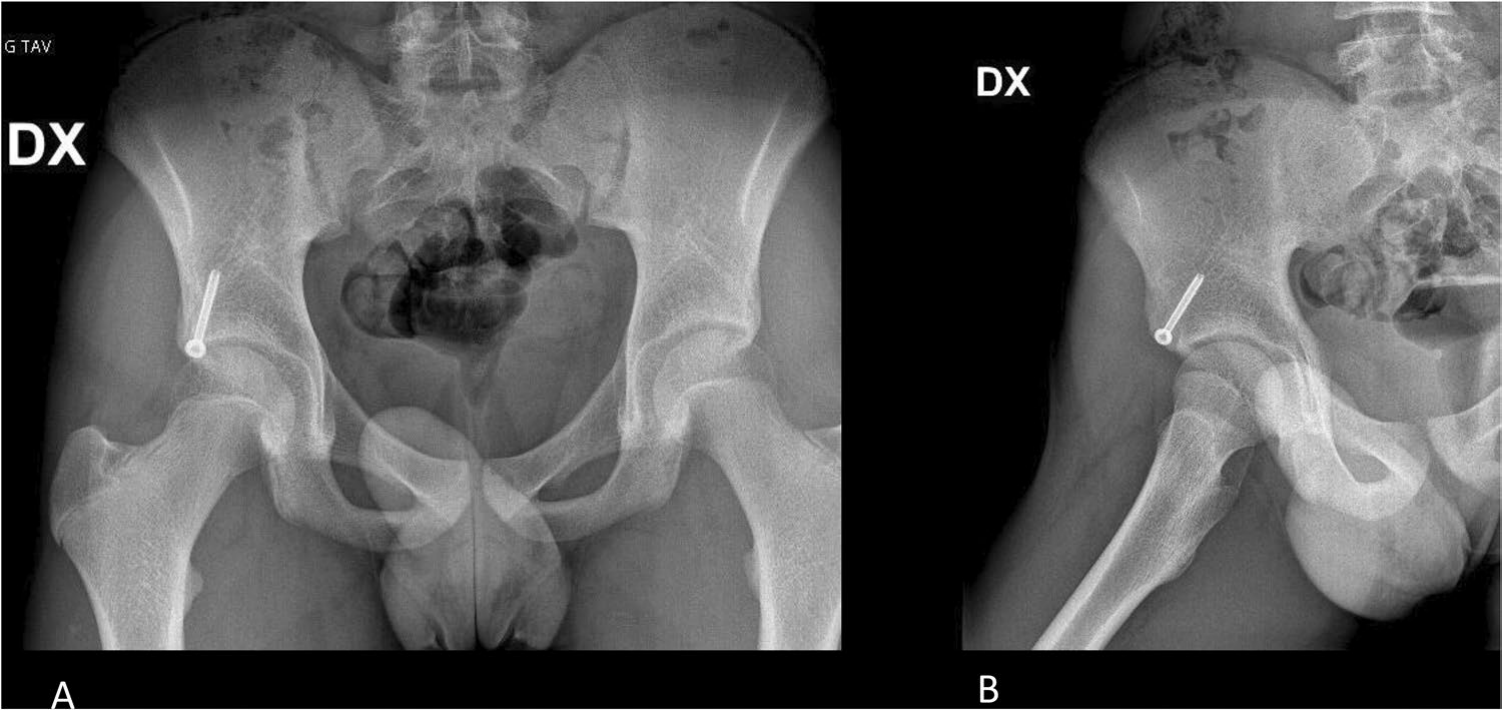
A, B One year follow up x-rays. A AP view, B Oblique view
